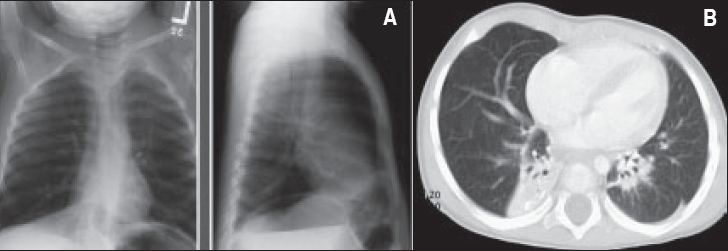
A patient with Spastic diplegia, brain atrophy, respiratory syncytial virus infection, gastroesophageal reflux and bilateral bronchiectasis. A- Chest X-ray: Increase density in lower lobes bilaterally. B. CT chest: Dilatation of bronchi of both lower lobes with collapse/ consolidation of affected lobes.
